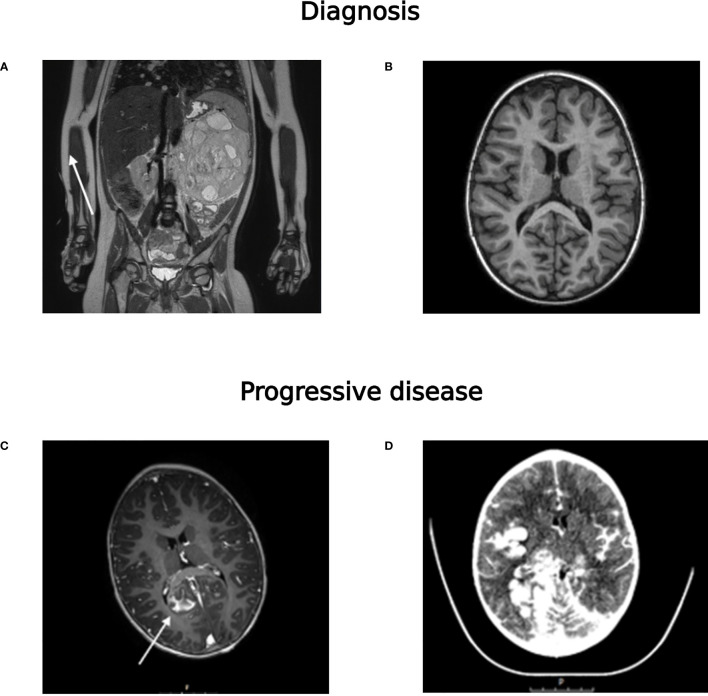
(A) MRI-scan (T2-TSE) at diagnosis showing the abdominal tumor, pulmonary metastases (indicated with an arrow) and a metastasis in the right proximal femur (indicated with an arrow), (B) MRI-scan of the brain (T1) at diagnosis, (C) after start of neurological symptoms (T1-gadolinium), showing extensive leptomeningeal metastases (indicated with an arrow) and (D) CT-scan (with ultravist contrast) shortly before death of the patient.
